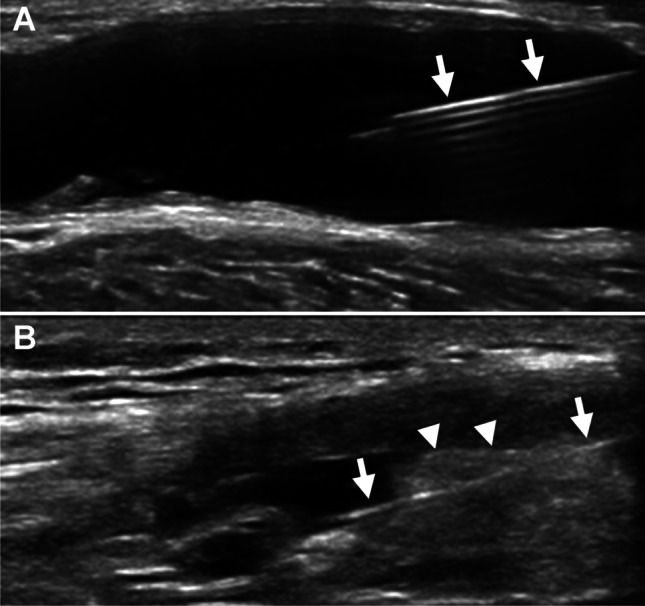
Ultrasound of (A) the puncture of a large Baker’s cyst and (B) the puncture of a knee joint from lateral into the suprapatellar recess with the whole needle in view (arrows), here penetrating a thick synovial fold (arrowheads) (images courtesy of Dr. B. Boddenberg-Pätzold, Cologne/Germany)
